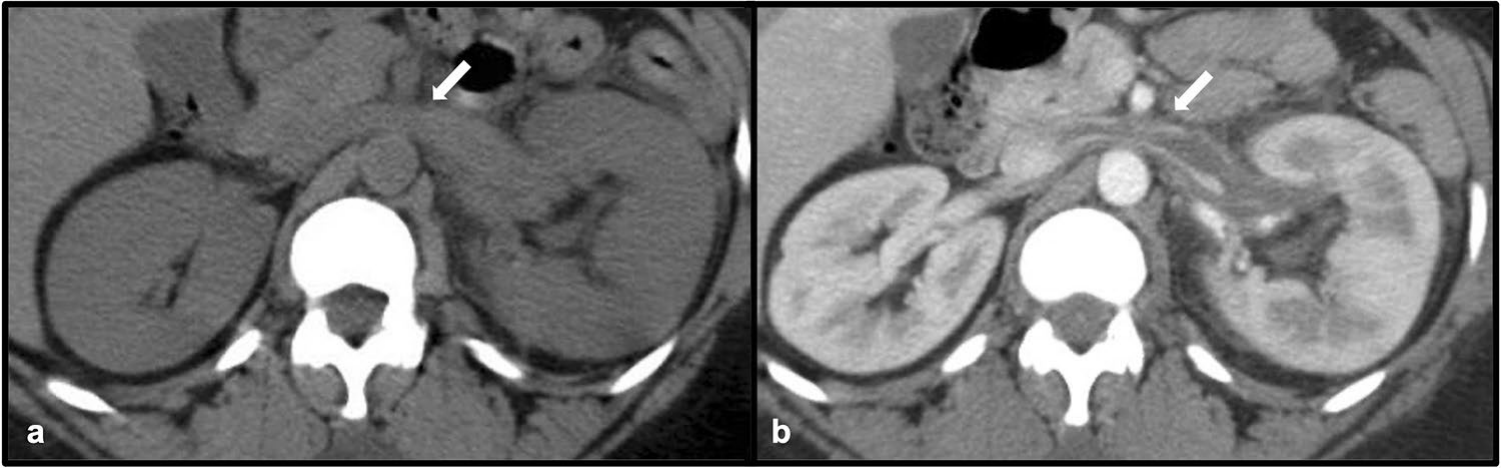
28-year-old male with nephrotic syndrome. Axial non-contrast CT of the abdomen (a) demonstrates expansion and hyperattenuation of the left renal vein (white arrow), as well as asymmetric enlargement of the left kidney. Axial contrast-enhanced CT (b) demonstrates a filling defect in the left renal vein consistent with renal vein thrombosis
